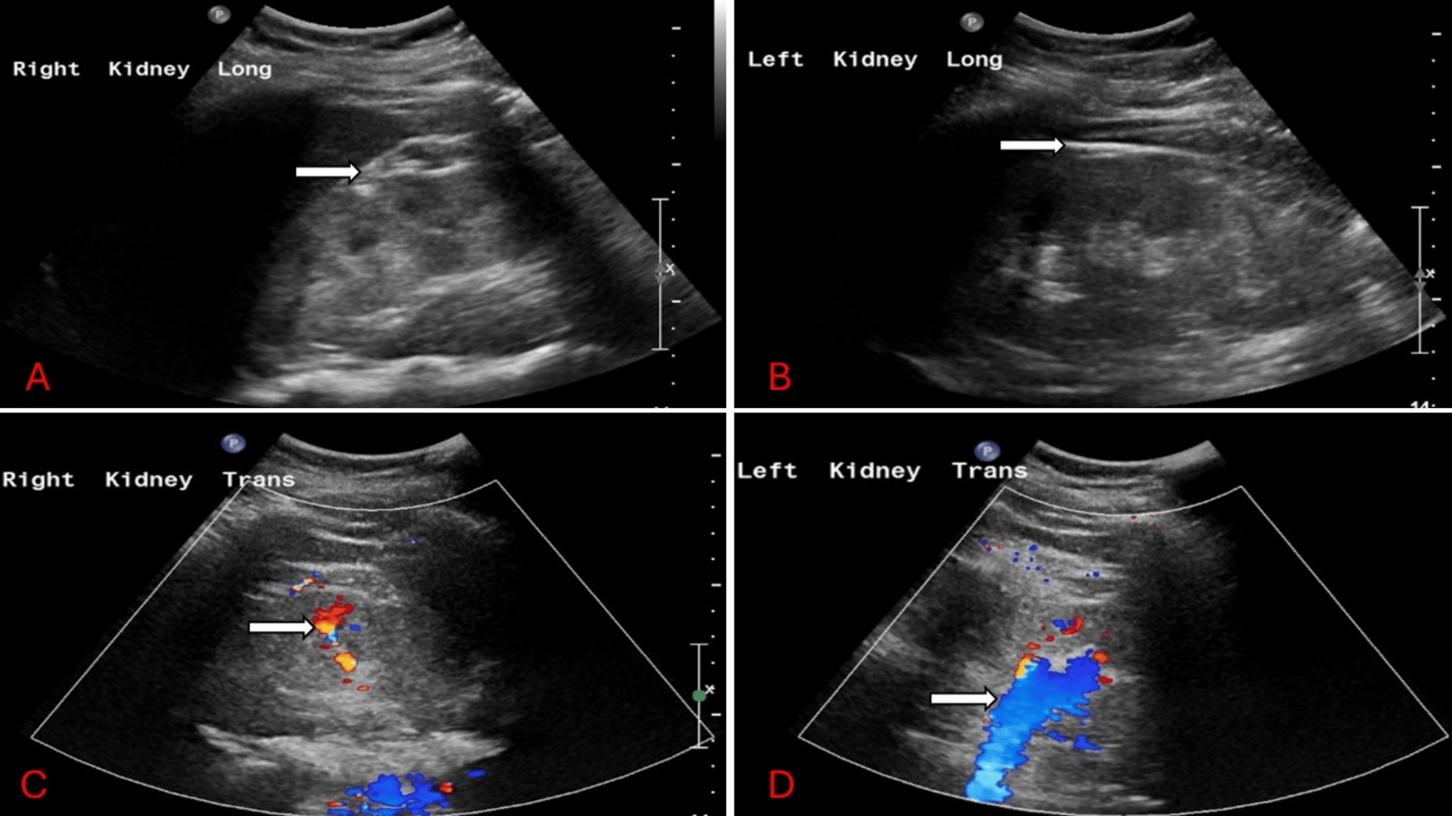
Ultrasound of patient's kidneys A: The right kidney measures 11.5 cm x 6.1 cm x 5.9 cm with increased echogenicity (arrow) but normal parenchyma thickness and contour and no pelvicalyceal dilatation, calculi, cysts, or solid masses. B: The left kidney measures 12.1 cm x 6.1 cm x 6.3 cm with increased echogenicity with corticomedullary differentiation (arrow) but normal parenchyma thickness and contour and no pelvicalyceal dilatation, calculi, cysts, or solid masses. C: Doppler ultrasound (arrow) of the right kidney. D: Doppler ultrasound (arrow) of the left kidney.
